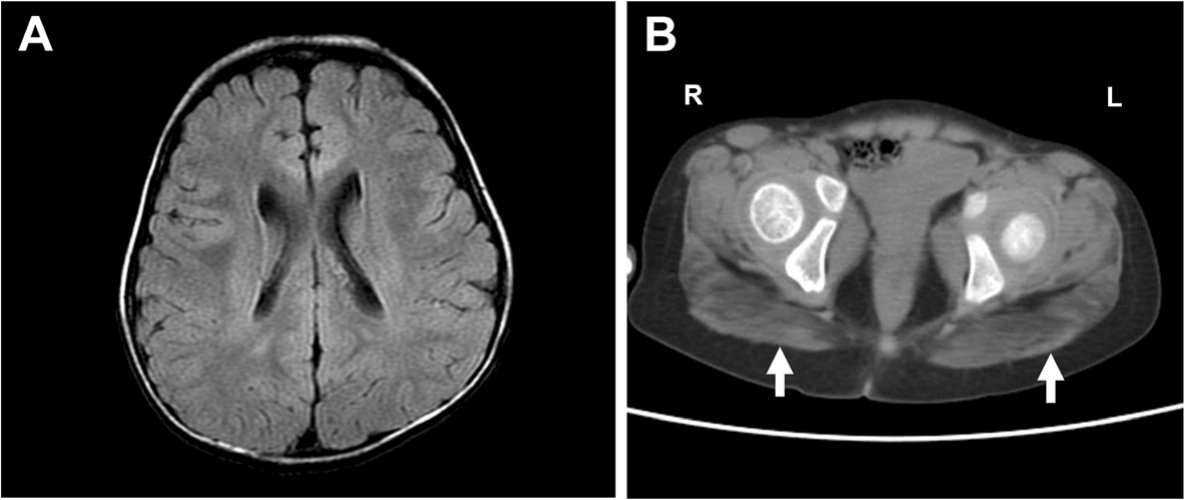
Brain and muscle imaging. a Mildly reduced periventricular white matter volume with angular-shaped ventricles was shown on brain MRI (T2-weighted flair). b Gluteal muscles were most affected on muscle CT as the arrows indicate. (*R* right, *L* left)
